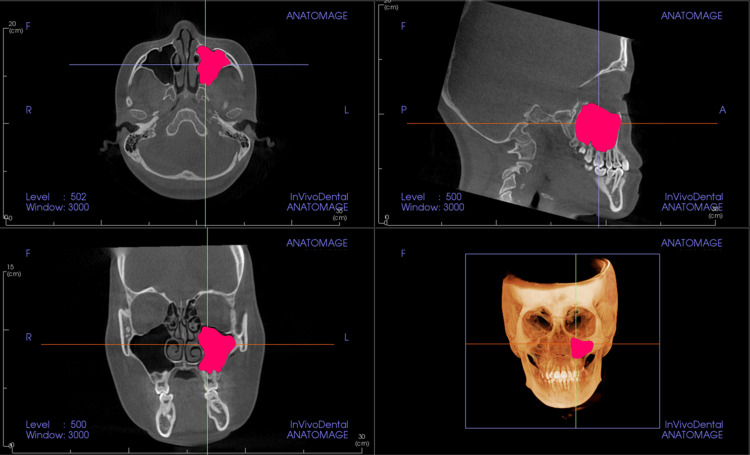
3D volume reconstruction of the maxillary sinus using cone-beam computed tomography images. The pink area represents the left maxillary sinus. Maxillary sinus volume measurements were taken for both the left and right sides.
